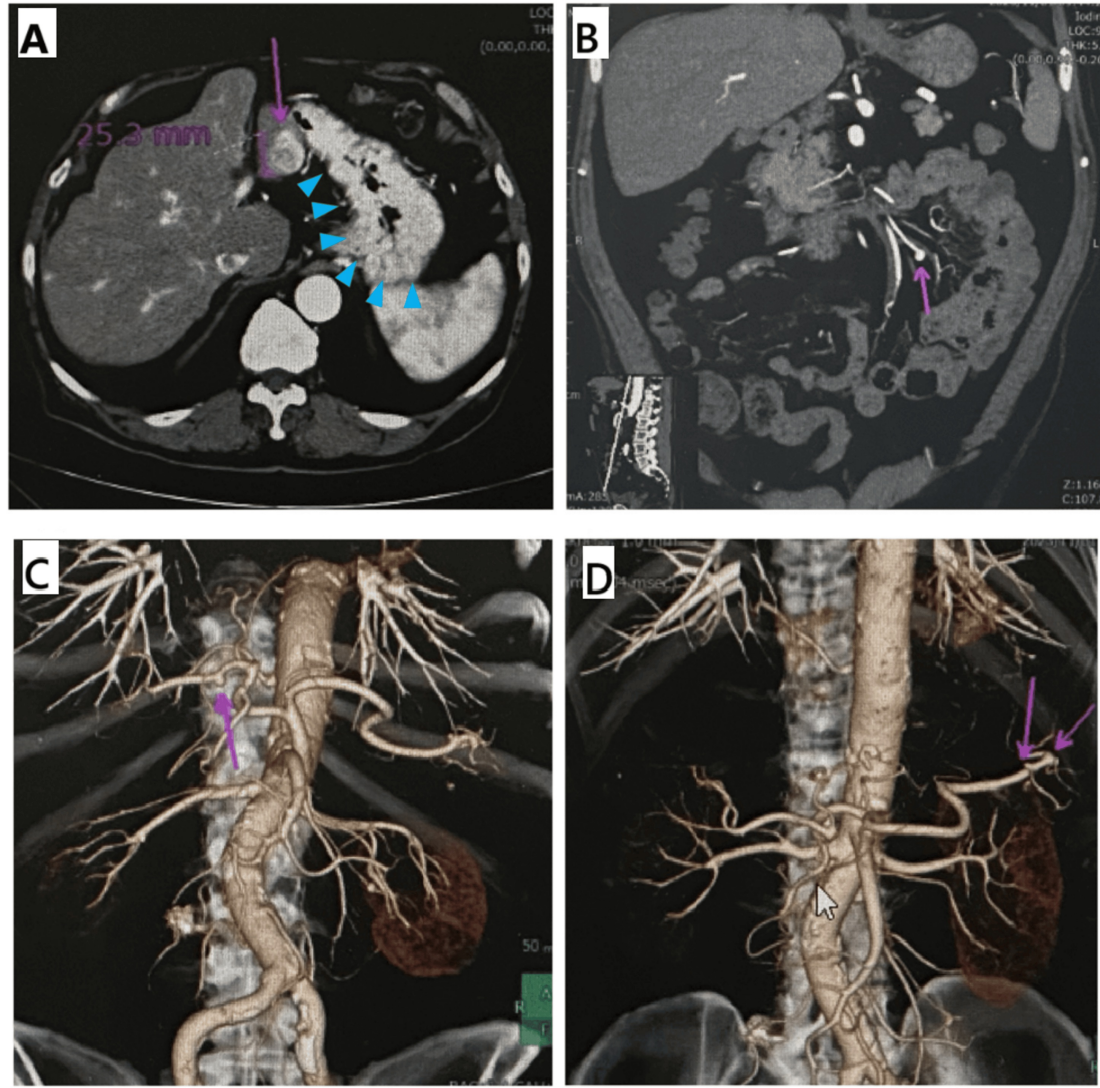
Preoperative CT images demonstrating HCC and vascular anomalies. (A) A 25-mm early enhancing lesion in liver segment 3 suggestive of HCC (purple arrow) and gastric varices (blue arrowheads). (B) Mesenteric arterial aneurysm (purple arrow). (C) Right hepatic arterial aneurysm (purple arrow). (D) Multiple aneurysms in the distal arterial trunk (purple arrows). These images demonstrate the extensive vascular abnormalities associated with the patient's portal hypertension HCC: hepatocellular carcinoma
